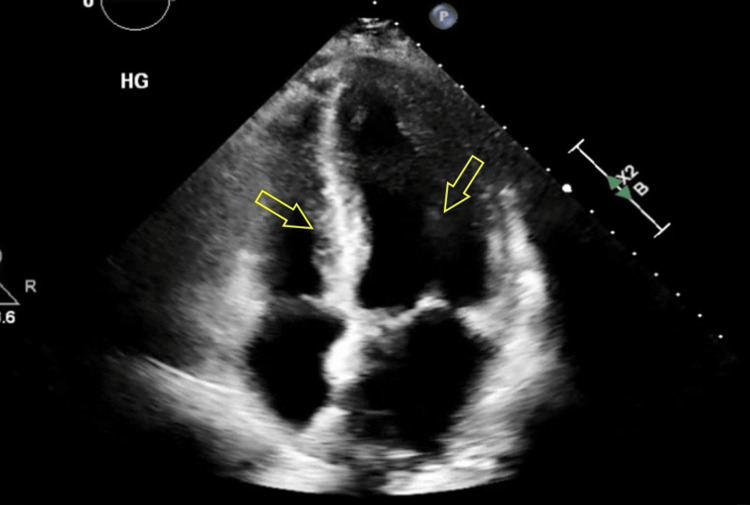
Echocardiographic findings suggestive of cardiac amyloidosis Transthoracic echocardiogram, apical four-chamber view, showing increased left ventricular wall thickness (arrows), suggestive of cardiac amyloidosis.
